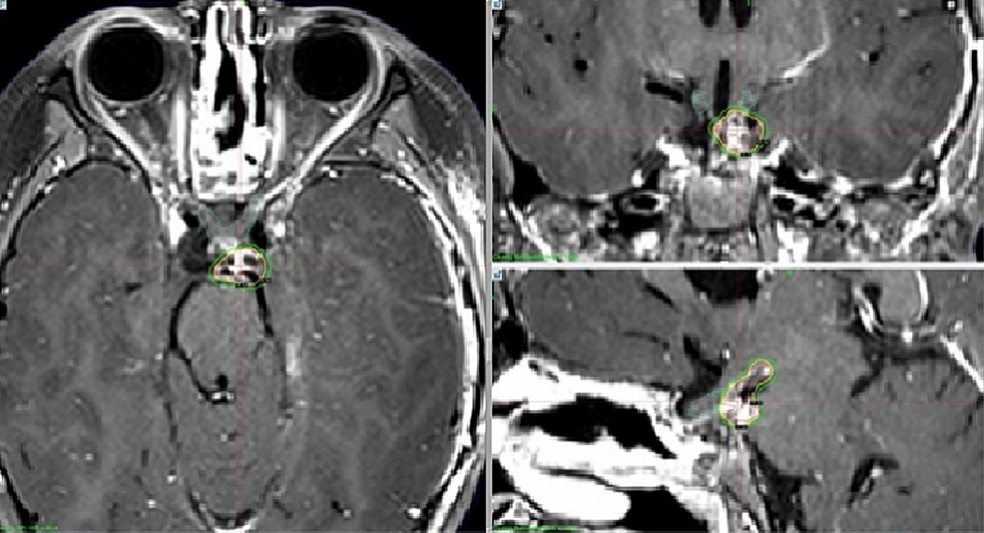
Case 3 Magnetic resonance images of a five-year-old girl with recurrent craniopharyngioma. A small suprasellar tumor was treated with the prescribed dose of 13 Gy at the tumor margin. Irradiation took 76.8 minutes.
